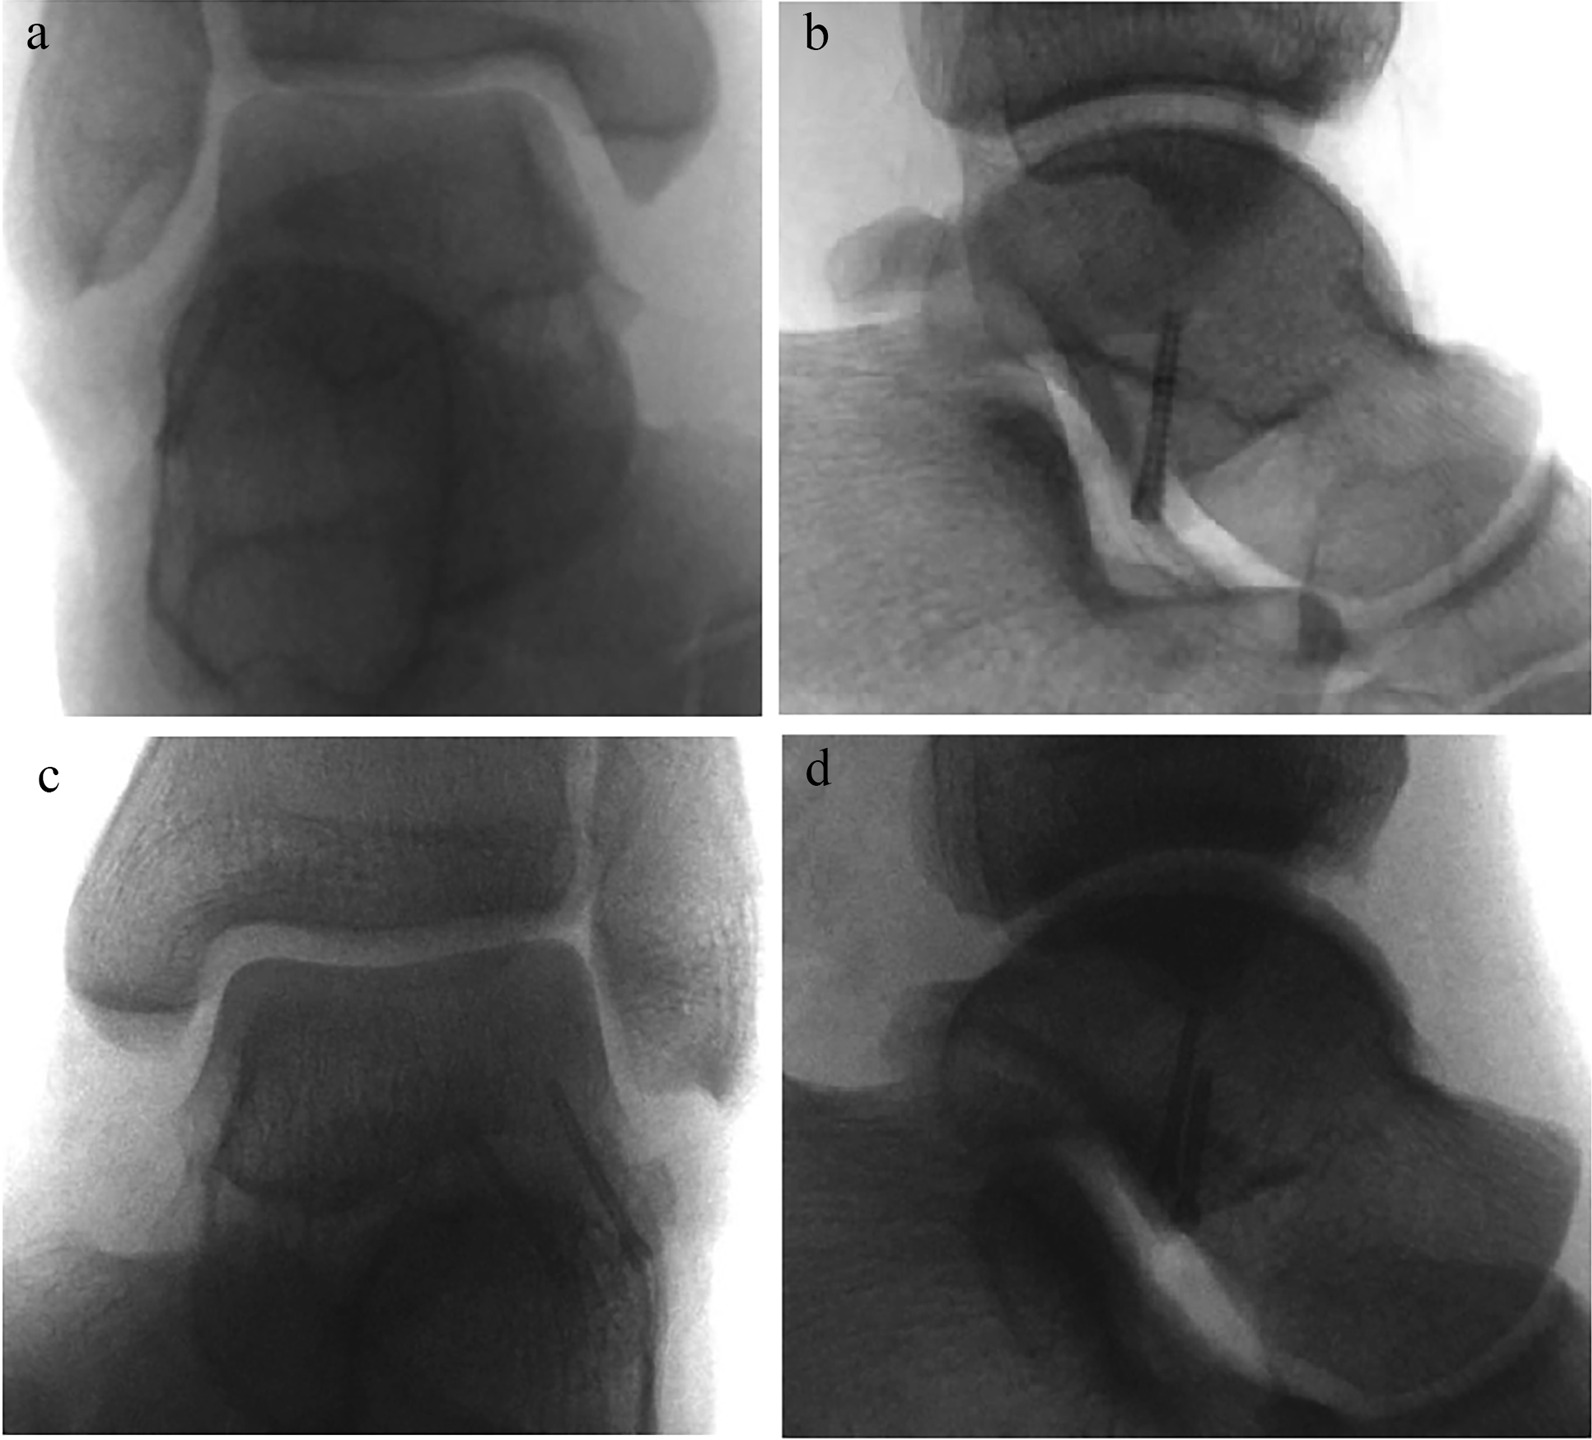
Intraoperative fluoroscopic images of the two cases after screw fixation of the lateral process of talus. Case 1: (a) Anteroposterior and (b) lateral radiograph showed screw fixation with one headless compression screw. Case 2: (c) Anteroposterior and (d) lateral radiograph showed screw fixation with two headless compression screws
